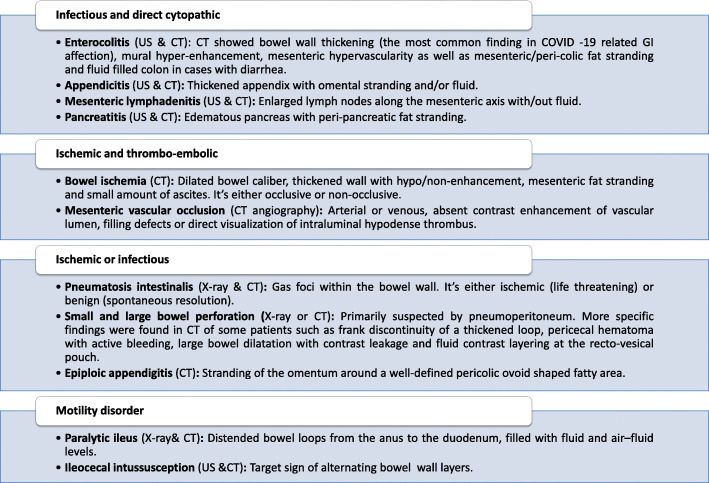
Abdominal radiological findings of COVID-19-associated GI symptoms. CT, computed tomography; US, ultrasound; GI, gastrointestinal
